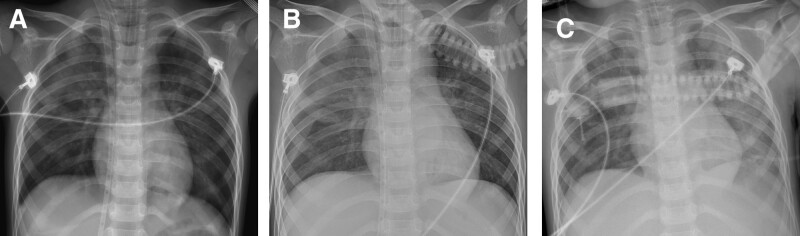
X-ray: The first day of the course of disease: pulmonary edema (A); The 4th d: pulmonary edema are slightly improved compared to the previous film, with the possibility of incomplete expansion of the right upper lung and pleural effusion on the right side (B); The 16th d: the lesions in both lungs are more severe than on the previous film, and there may be fluid accumulation in the left pleural cavity (C).
